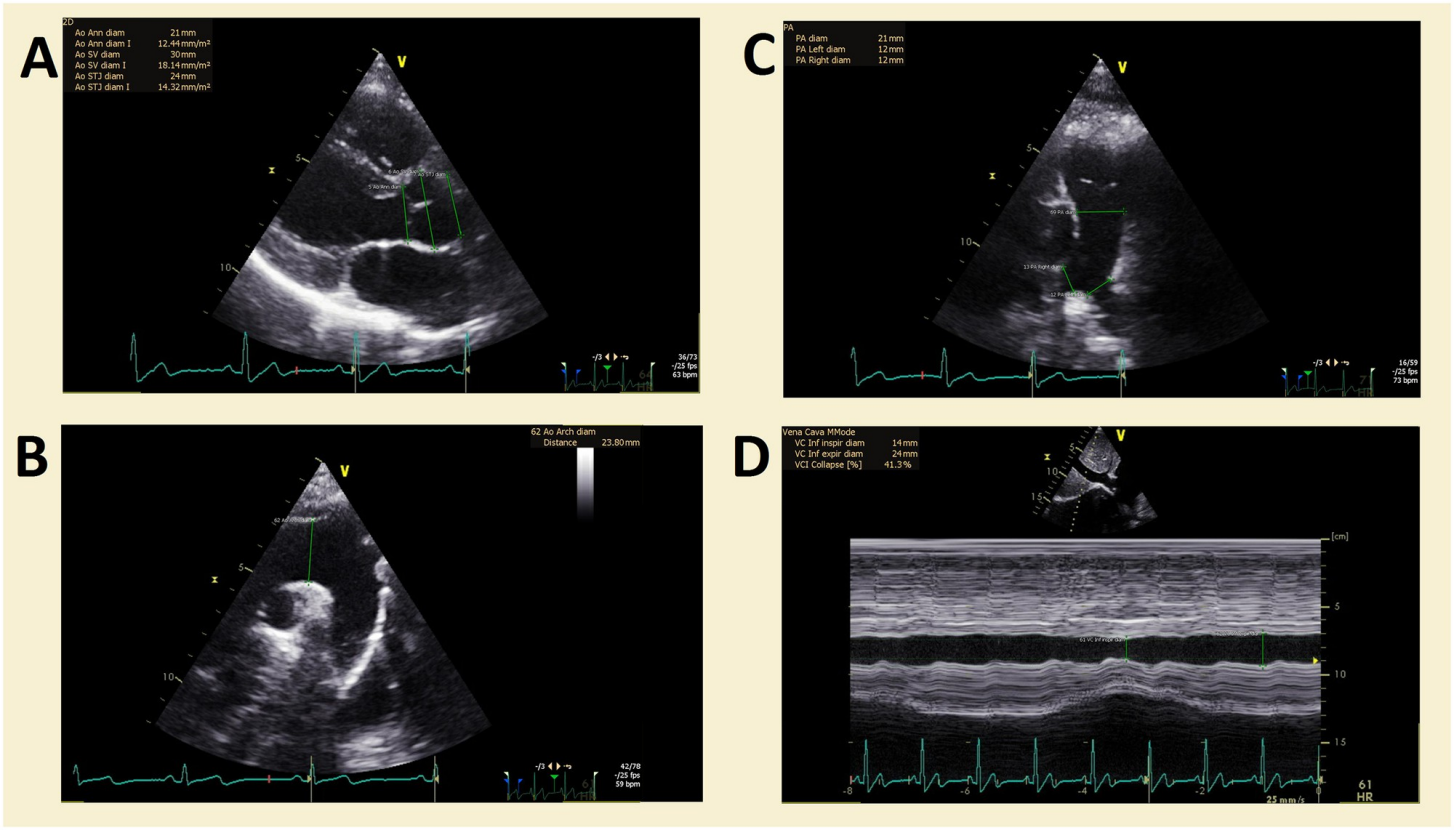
An example of echocardiographic measurements for the study. A–measurements of the aorta in the parasternal long axis (aortic annulus, sinus of Valsalva and sinotubular junction diameters). B–measurement of the arch of the aorta in the suprasternal view. C–measurement of the diameter of the main pulmonary artery and the left and right pulmonary arteries in the modified parasternal short axis with focus on the main pulmonary artery. D–measurement of the inferior vena cava during inspiration and expiration–measured in M-mode.
